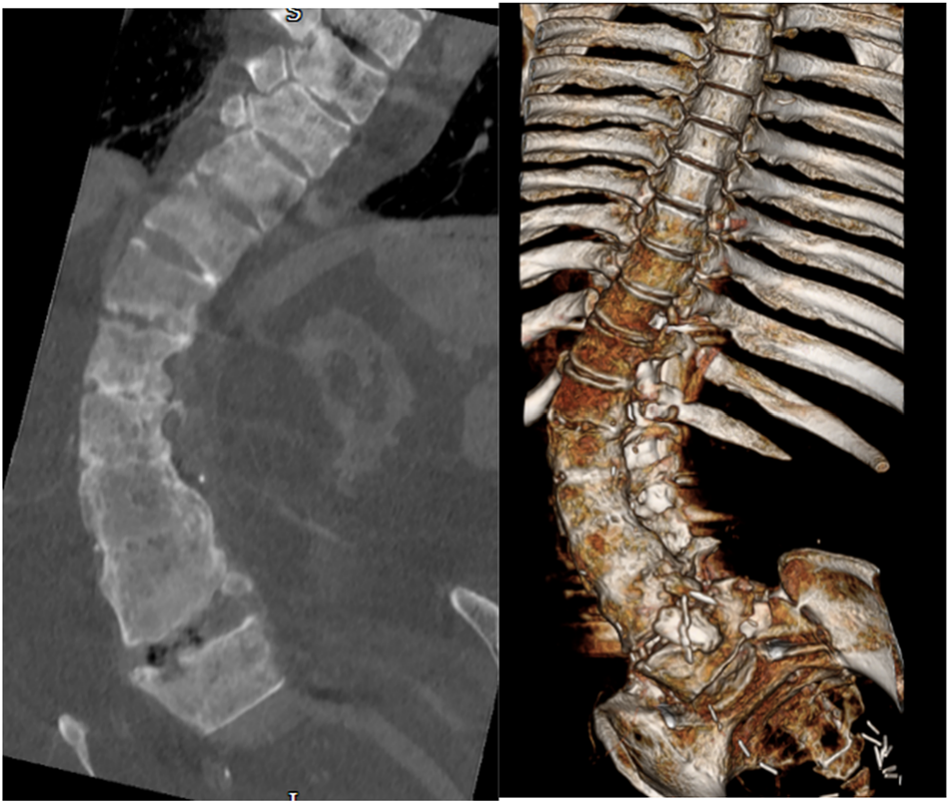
Coronal view of thoracic and lumbar spinal CT: a break in the fusion mass at the L4/L5 level is noted, where there is vacuum phenomenon and bony irregularity within the disc space locally. There is also a mature appearing cleft/deficit within the posterior elements at this level. The appearances locally are suggestive of a pseudoarthrosis secondary to failure at the lower end of the effusion. Bony overgrowth is leading to modelling abnormality and canal deformity.
